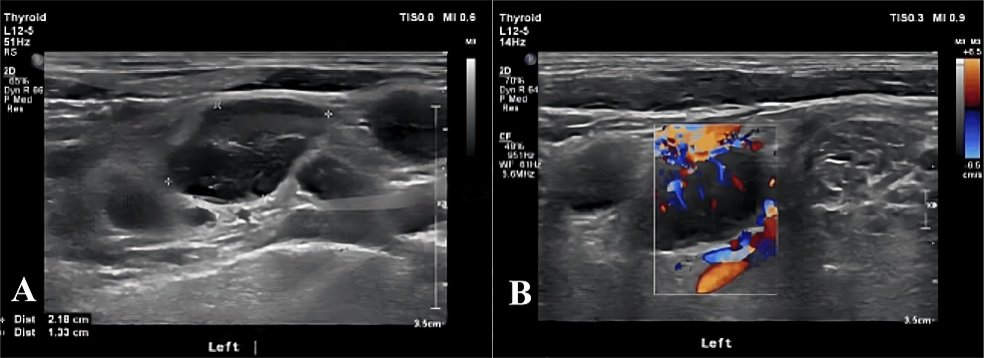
Ultrasound of lymph nodes Ultrasound of lymph nodes in the neck and supraclavicular fossa identified several hypoechoic lymph nodes, with the larger ones measuring 2.2×1.3cm and displaying an unclear corticomedullary boundary.
